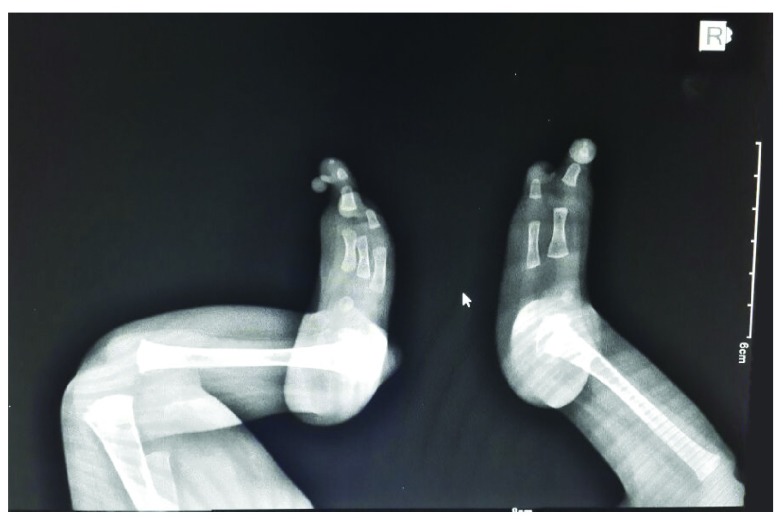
X-ray showing absence of right 3,4,5 metatarsals and phalanges, absence of left 4,5 metatarsals and phalanges.
